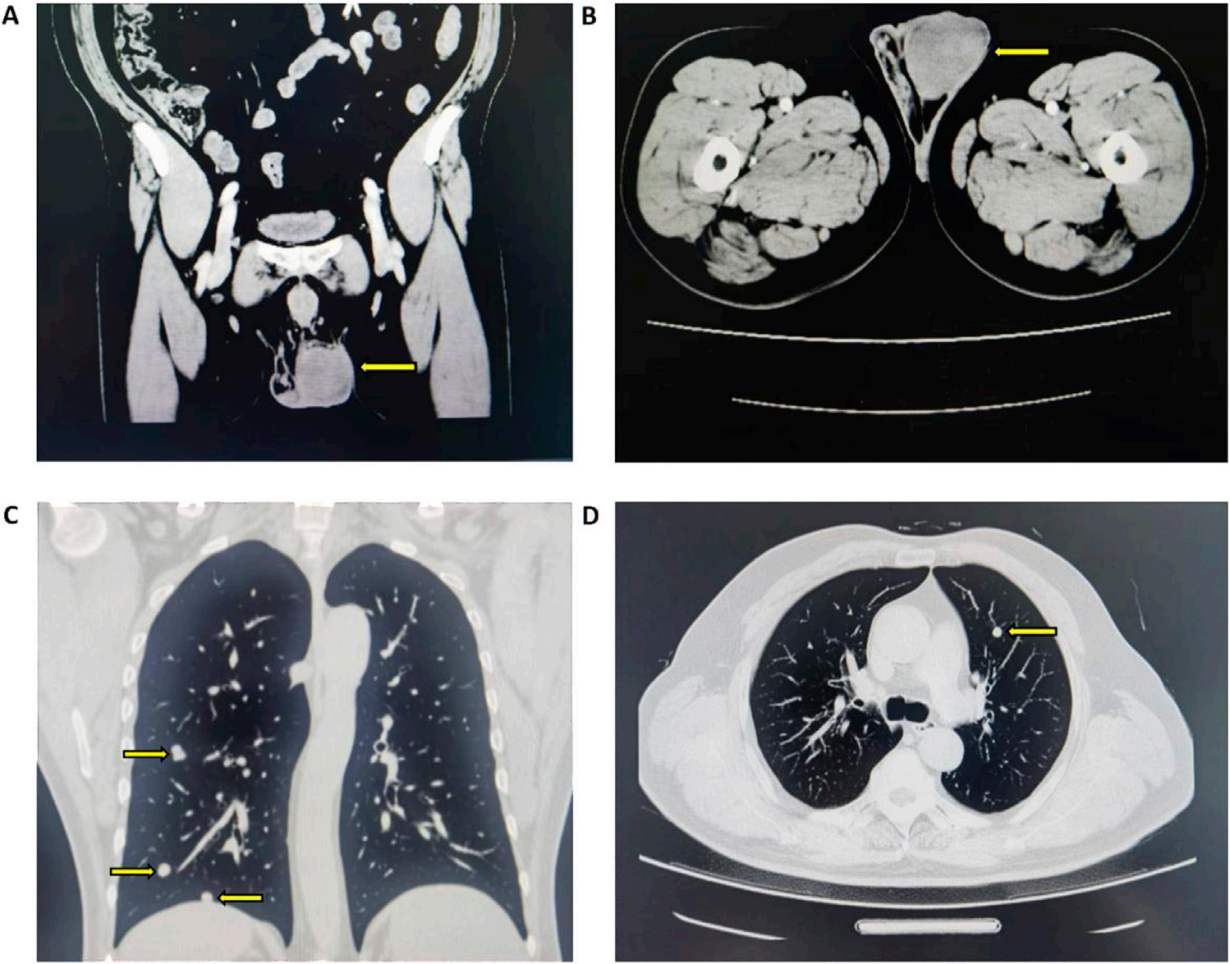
CT imaging findings of left testicular neoplasm and bilateral pulmonary nodules (A) Coronal view of left testicular mass-like slightly hyperdense focus with mild enhancement (B) Axial view of corresponding left testicular lesion (C) Coronal view of bilateral pulmonary nodules (D) Axial view of bilateral pulmonary nodule.
